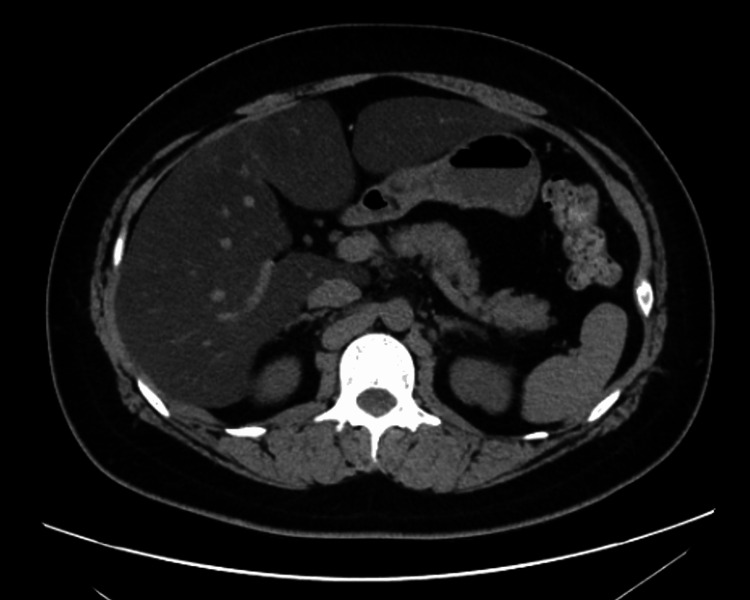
Abdominal plain CT findings upon admission. The liver demonstrates lower attenuation compared to the spleen. The characteristic feature of acute pancreatitis, which is the increase in peripancreatic fat density, is not clearly observed.
